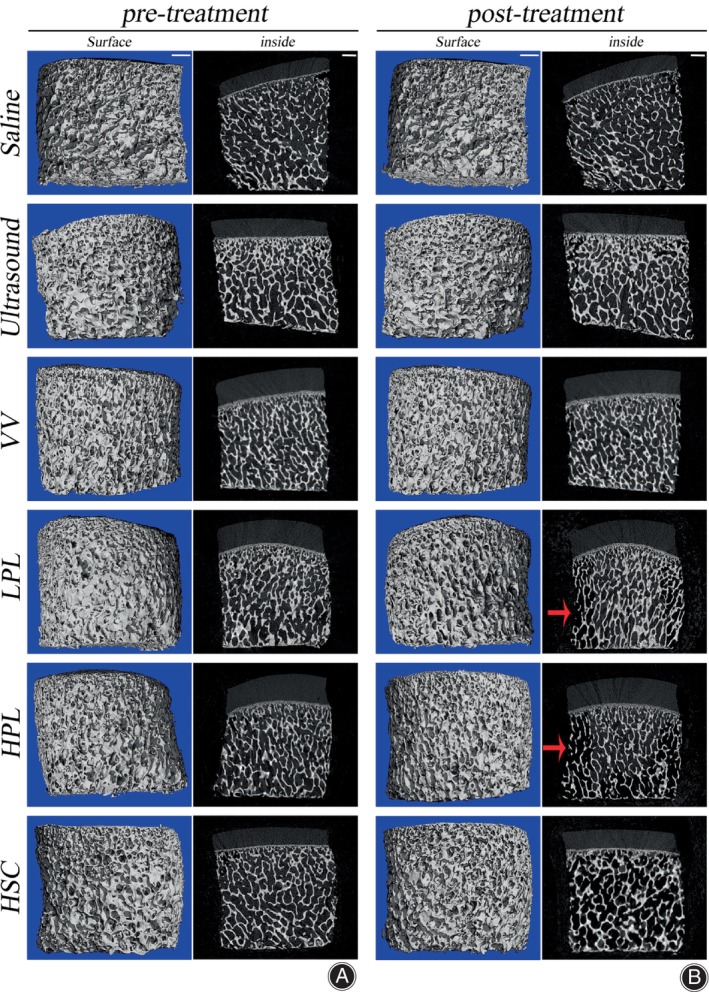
Micro‐Computed Tomography Evaluation of OCAs. (A) The 3D and 2D pictures of pre‐treatment OCAs. Left‐side scale bar = 1000 μm and right‐side scale bar = 1000 μm. (B) The 3D and 2D pictures of post‐treatment OCAs. The red arrows point to sparse bone trabeculae. Left‐side scale bar = 1000 μm and right‐side scale bar = 1000 μm. HPL, high‐pulse lavage; HSC, high‐speed centrifugation; LPL, low‐pulse lavage; OCAs, osteochondral allografts; VV, vortex vibration.
